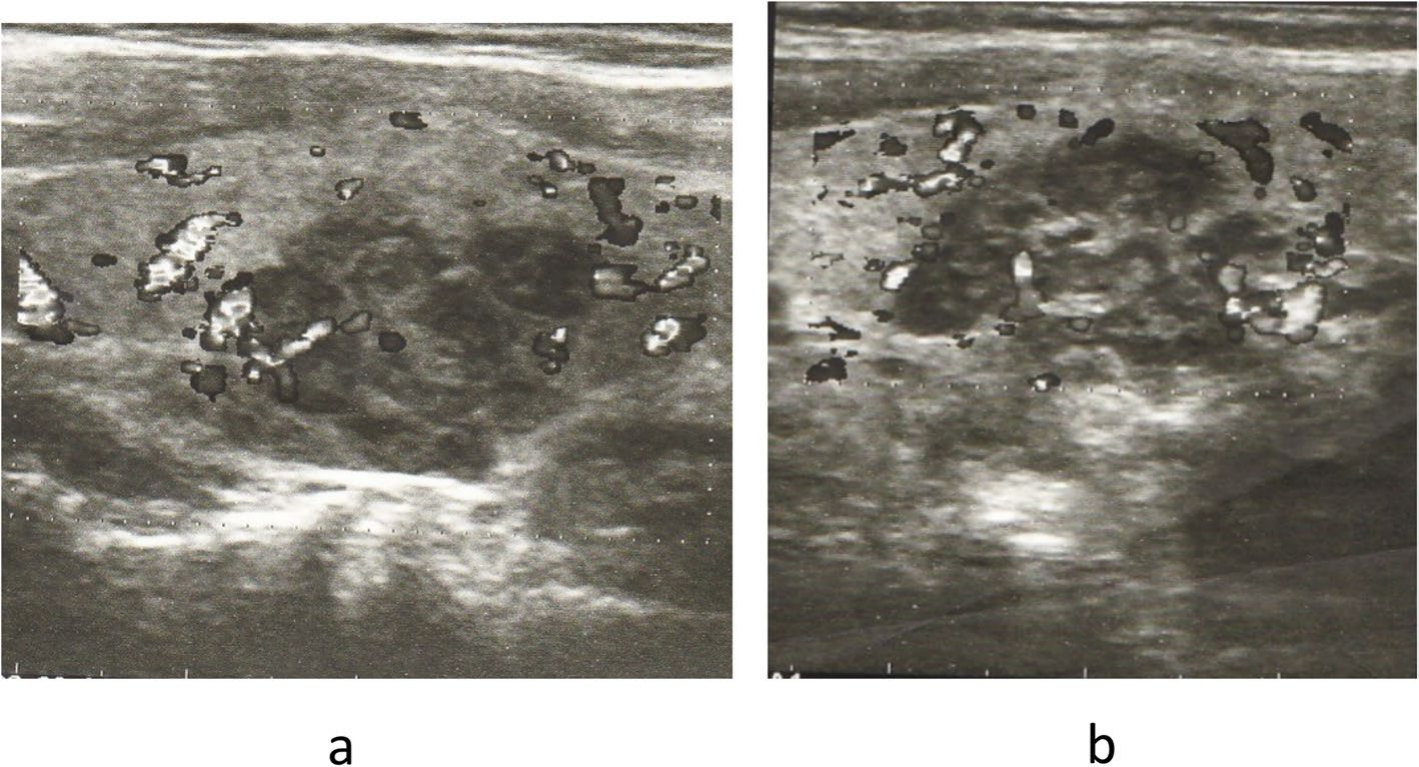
a Thyroid ultrasound. Right thyroid lobe with hypoechoic, perinodally vascularized nodule measuring 30 × 25 × 36 mm. b Thyroid ultrasound. Left thyroid lobe with hypervascularized, irregular hypoechoic area measuring 20 × 12 × 16 mm
